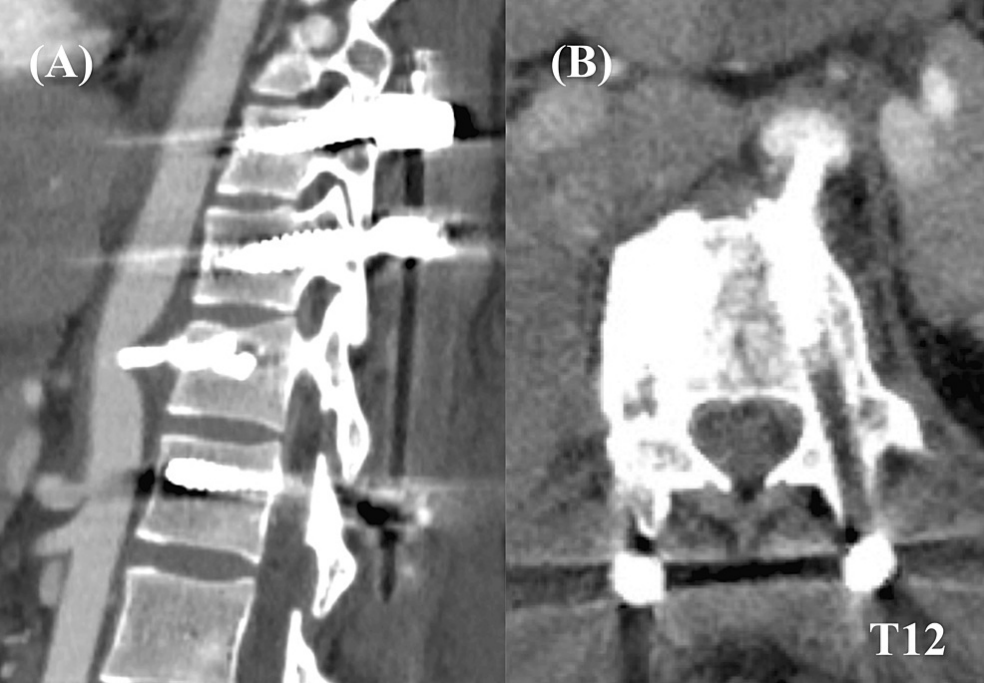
Postoperative contrast-enhanced computed tomography: (A) sagittal view and (B) axial view. HA blocks are observed compressing the descending aorta. HA, hydroxyapatite
